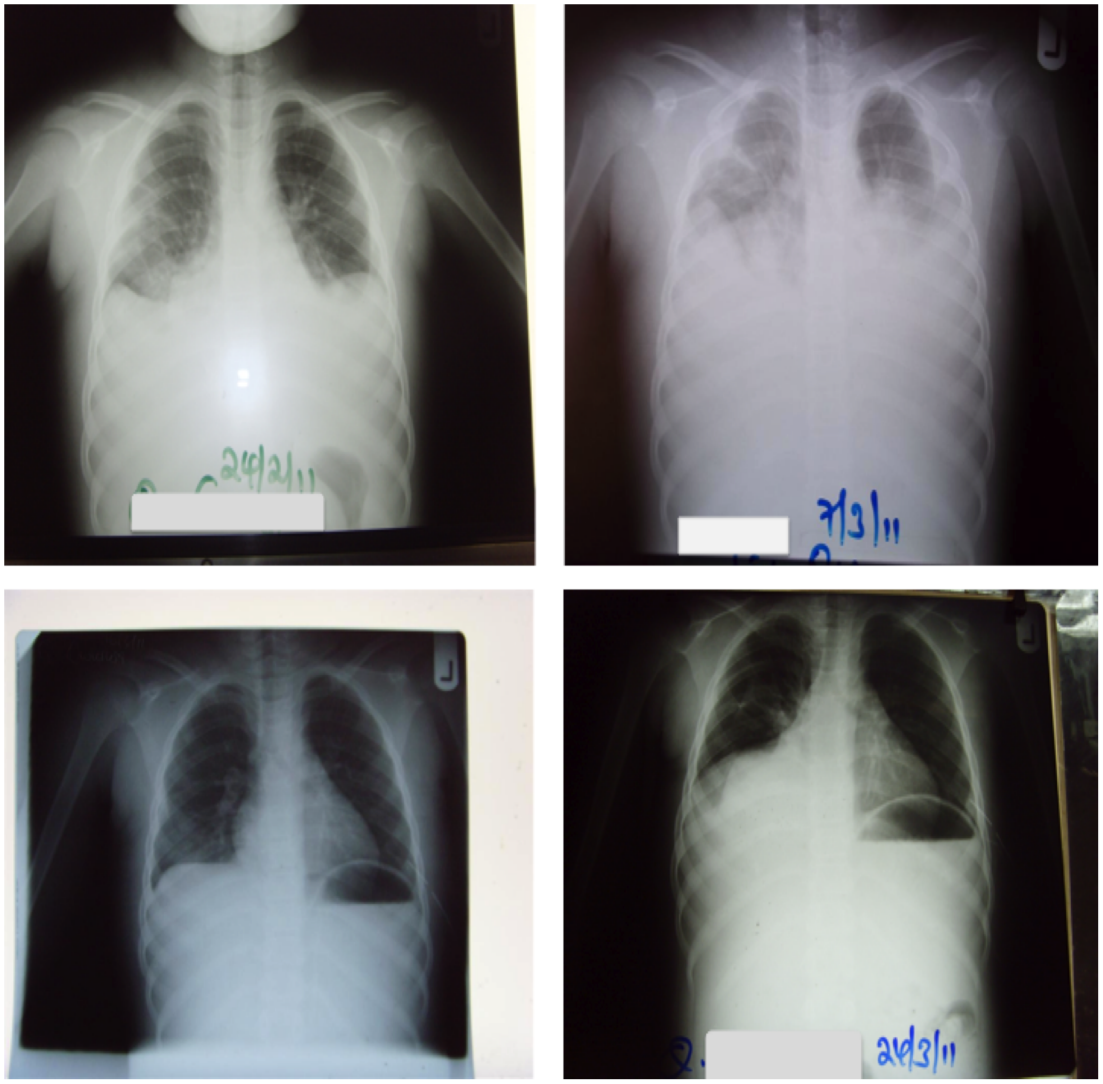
Chest radiographs of patient before and during antibiotic chemotherapy for *M. ulcerans* disease. (A) Chest X-ray taken before antibiotic therapy was started. (B) Chest X-ray taken 2 weeks after initiation of antibiotic therapy, showing bilateral pleural effusions. (C) Week 3 after drainage of pleural effusion. (D) Recurrence of pleural effusion on the right hemithorax at week 4.
